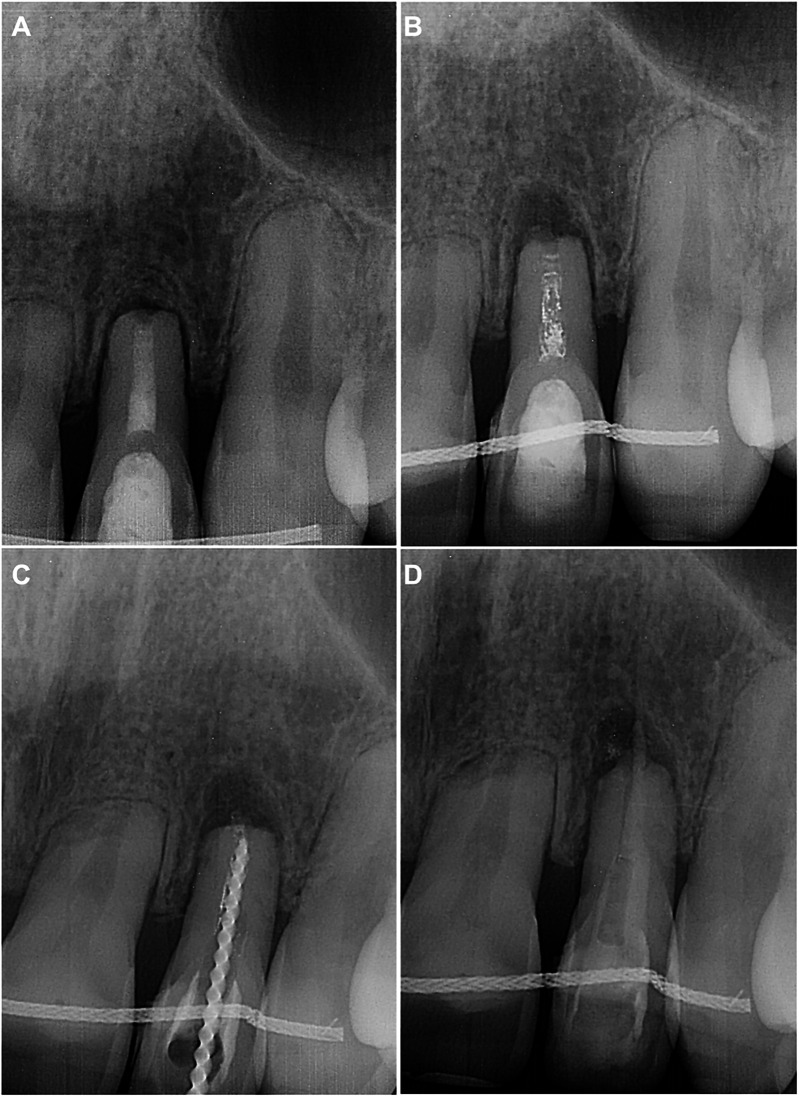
(A) Periapical radiograph performed during examination 2 weeks after the secondary dental trauma. (B) Eighteen-month follow-up periapical radiograph demonstrating changes in homogeneity of the root canal filling, along with a periapical lesion on tooth 22. (C) Intraoperative periapical radiograph for retreatment WL determination. (D) Intraoperative periapical radiograph after placing Biodentine in the apical and middle thirds of the root canal.
